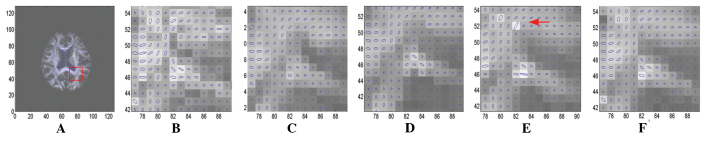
Visual assessment of the denoising effects on *in vivo* DTI data. The slice shown is the same as in Fig. 3 and 4, and each tensor is represented by an ellipsoid. (A) A slice of the *in vivo* dataset. (B) Enlarged view of the boxed region in (A). Denoising with (C) RD, (D) LED and (E) ED weighting and (F) UNLM in DWI space. DTI, diffusion tensor imaging; RD, Riemannian distance; LED, Log-Euclidean distance; ED, Euclidean distance; UNLM, unbiased non-local mean; DWI, diffusion-weighted image.
